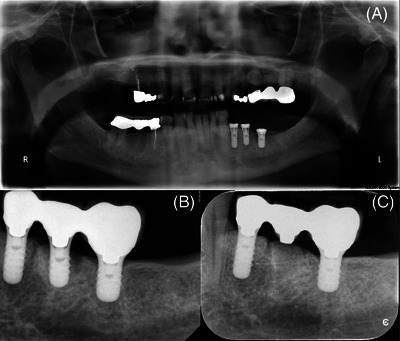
Radiographic series for patient 3. (A) a panoramic image showing the three implants (Straumann SP) placed at the left mandibular posterior sextant in 2011; (B) in January 2023, the middle implant was seen to have suffered severe bone loss and was considered a failure; (C) it was removed, its socket grafted and the modified FDP re‐inserted.
